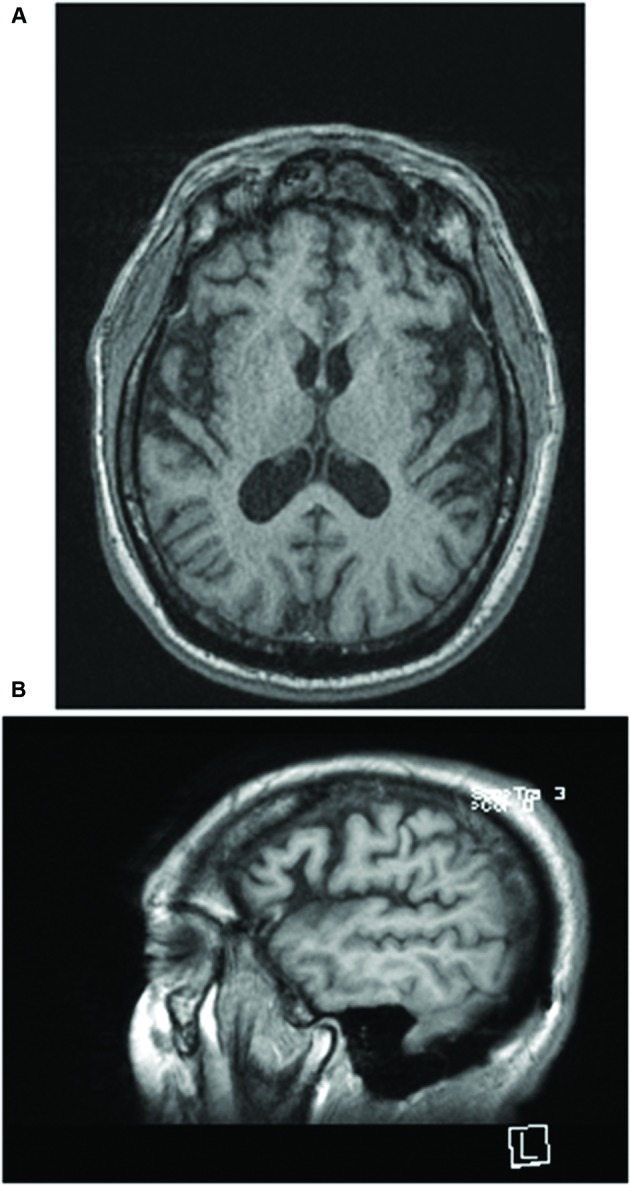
(A) T1 axial and (B) sagittal images of the brain obtained 9 months earlier demonstrate mild global volume loss for age with widening of the Sylvian fissure bilaterally. Neither evidence of past acute TBI nor white matter abnormalities were noted on fluid-attenuated inversion recovery (FLAIR) imaging.
